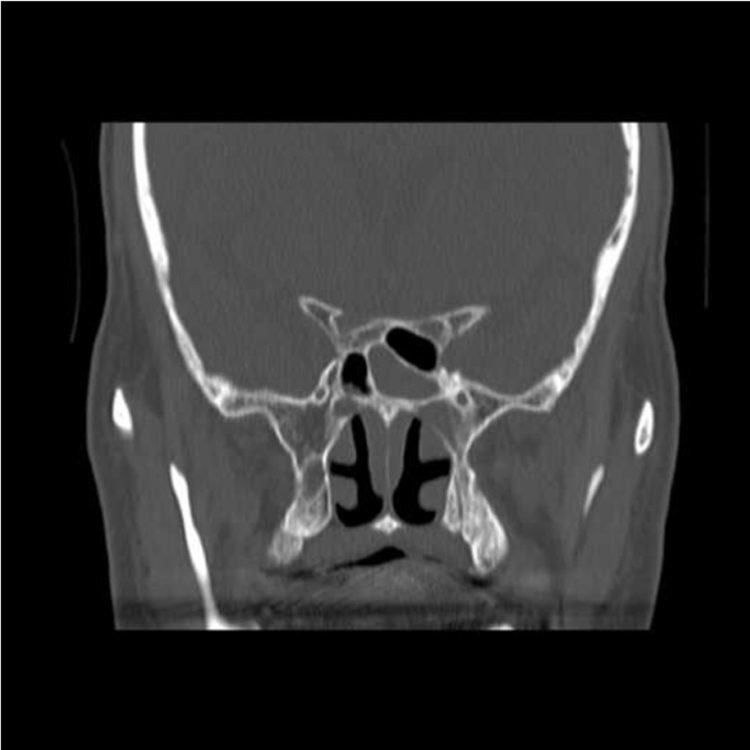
CT image showed fluid effusion in his left sphenoid sinus.
